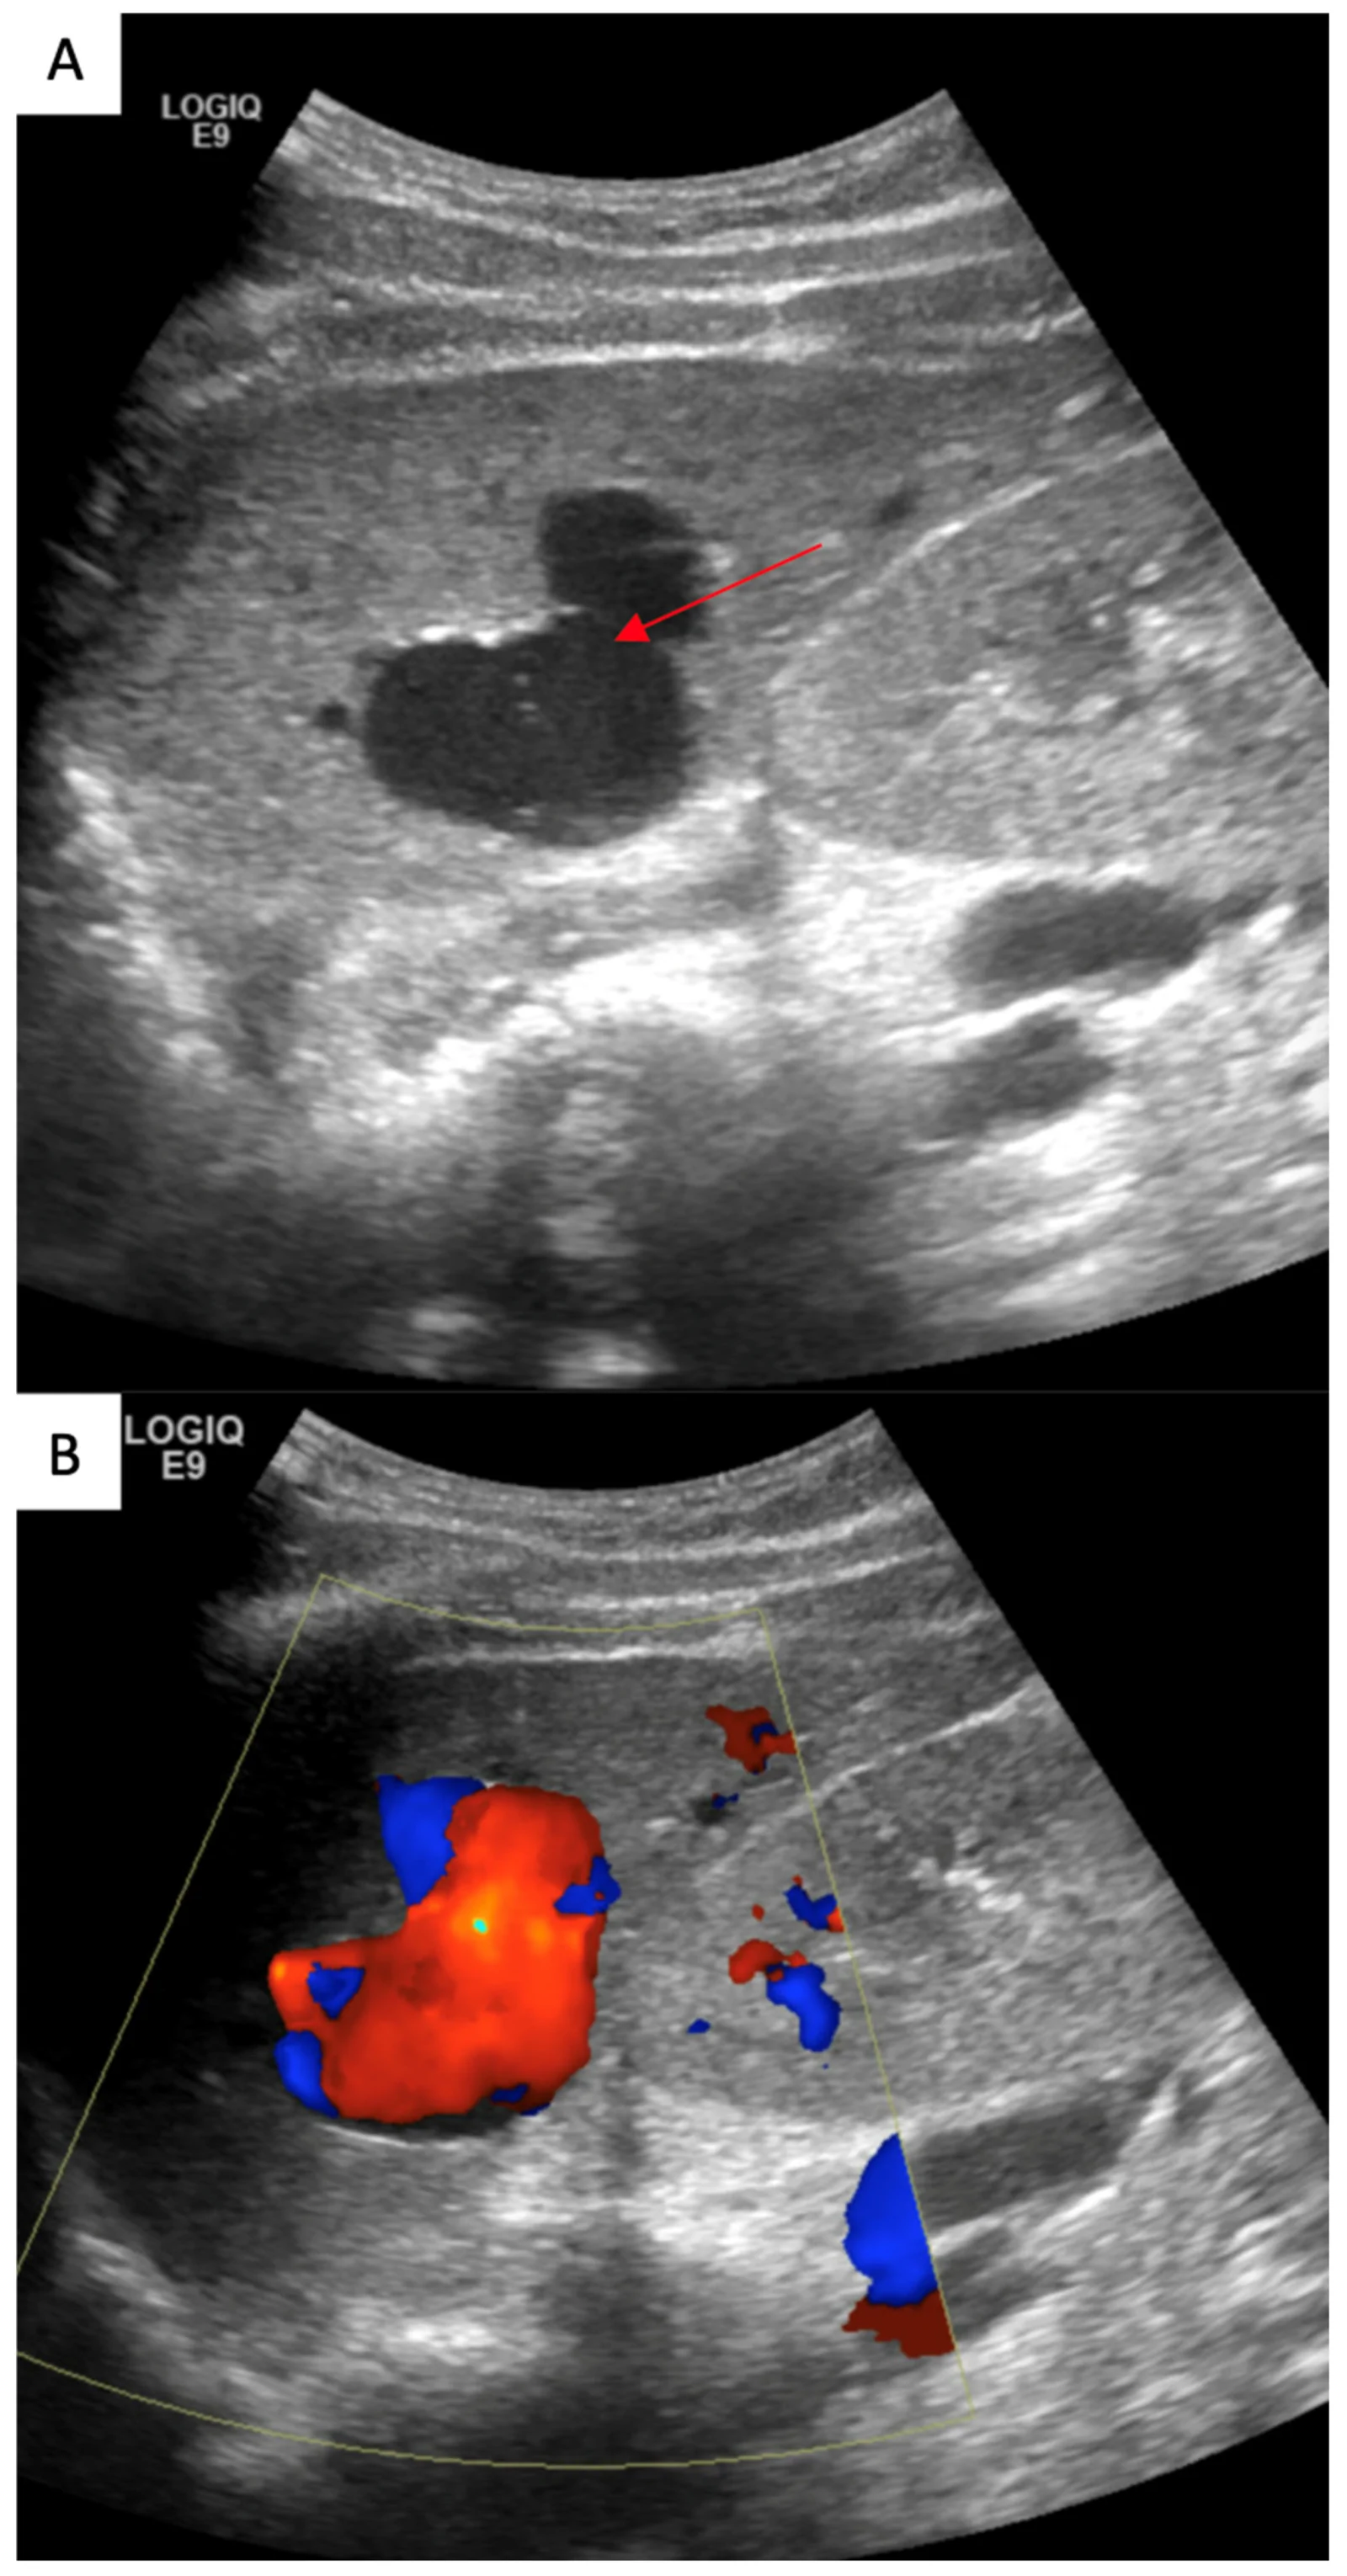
(A) Abdominal ultrasound, without Doppler, showing presence of an intrahepatic portosystemic venous shunt (arrow); (B) Abdominal ultrasound, with Doppler, showing presence of an intrahepatic portosystemic venous shunt.
